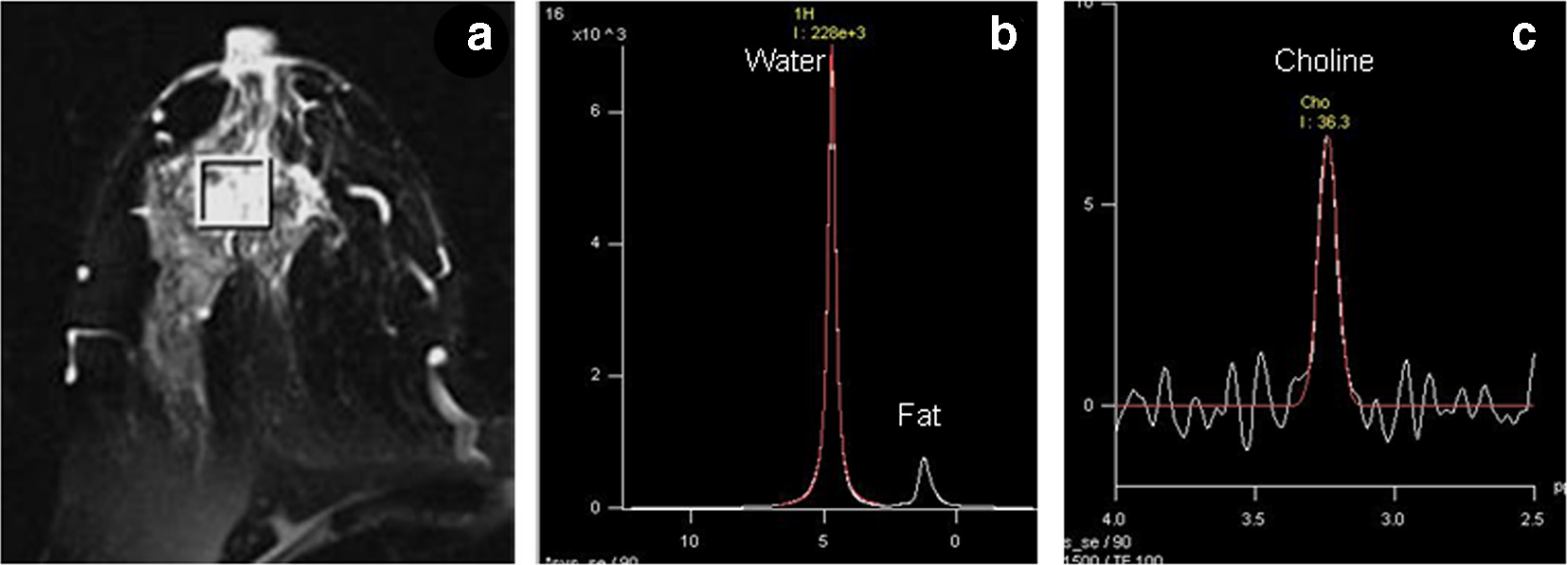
(a) *T_2_* weighted MR image of a patient suffering from locally advanced breast cancer while (b) shows the *in vivo*
